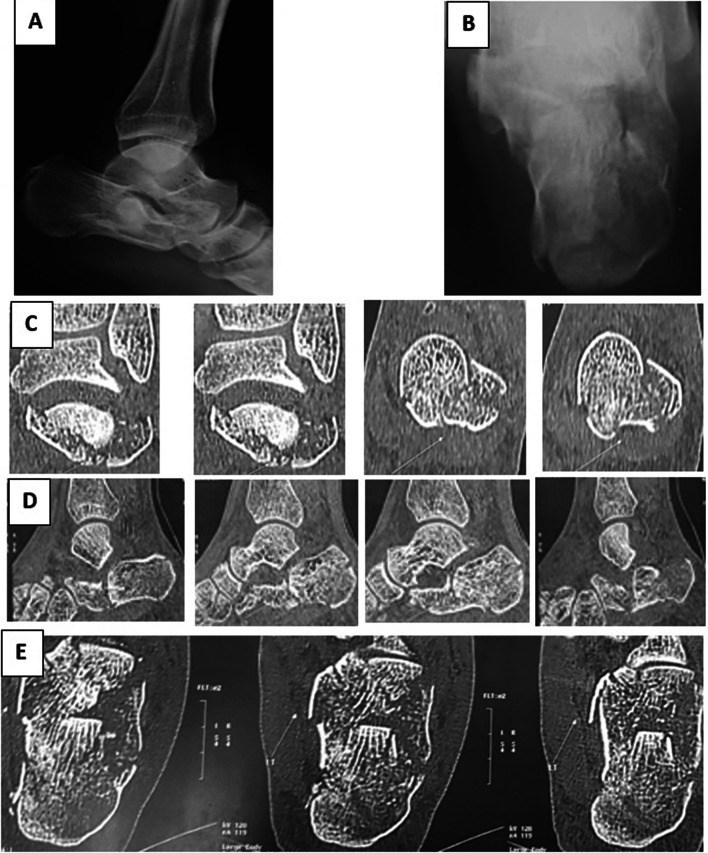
Complete case presentation. A, B Lateral and axial plain preoperative radiographs, respectively. C, D, E Coronal, sagittal and axial preoperative CT cuts
